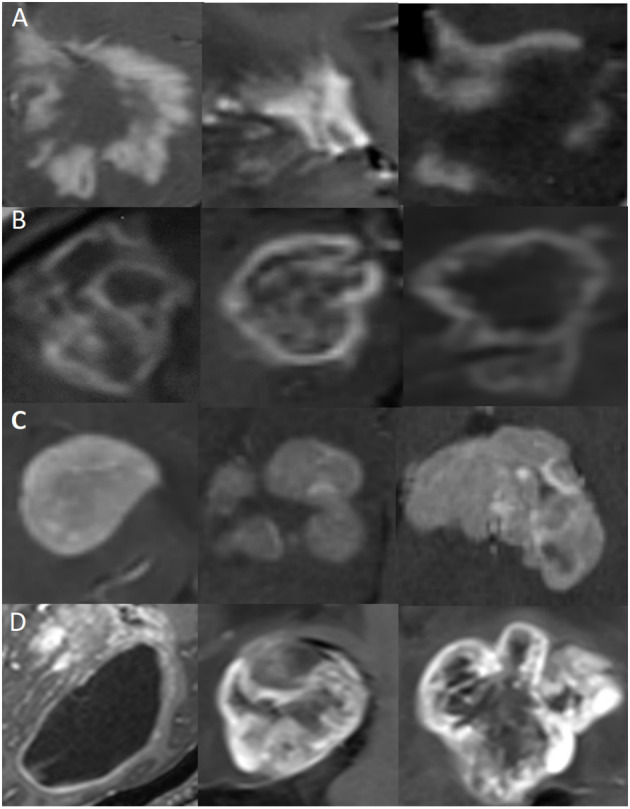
Representative MR images of clipped regions of interest (ROIs) for the four types of diseases. Each row represents a distinct disease, and each image is from a different patient. (A) ROIs from TDLs. (B) ROIs from TPACNS. (C) ROIs from PCNSL. (D) ROIs from gliomas.
